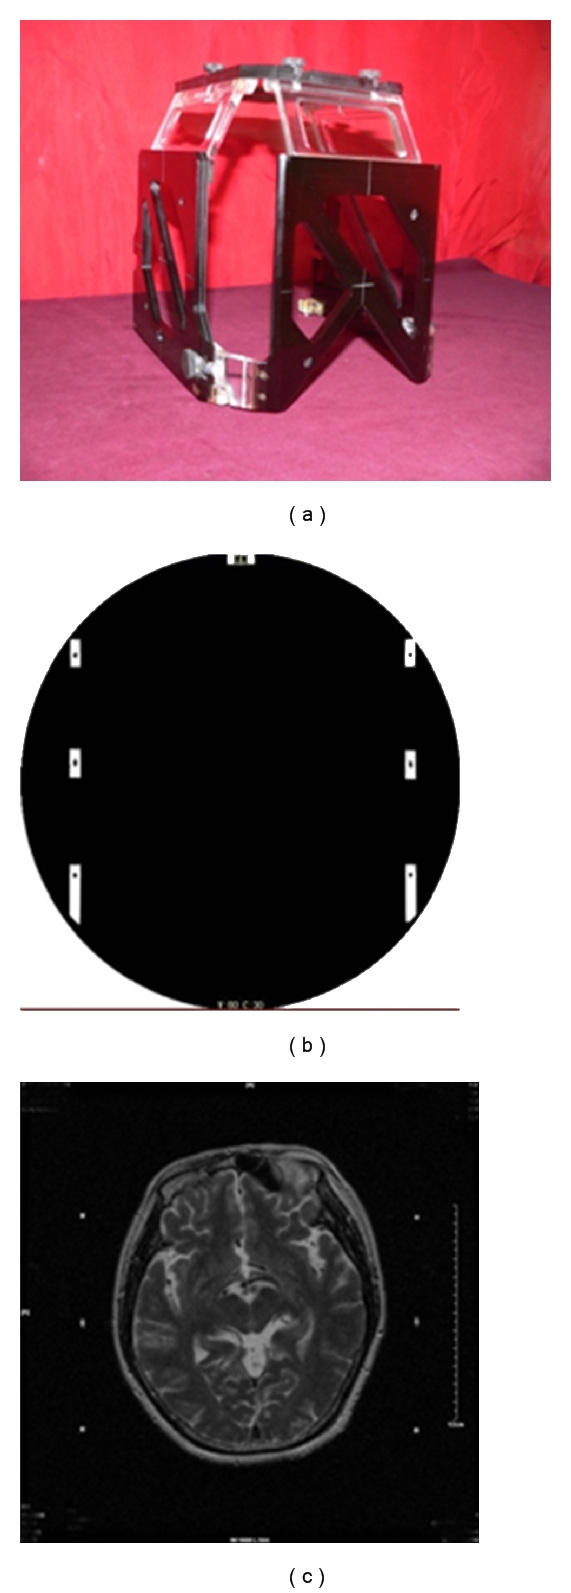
(a) A head frame with contrast agent of copper sulphate solution filled in the N shape frames. (b) A CT slice with the frame on. (c) An MR slice at the same head position (same z direction) as that in the CT.
